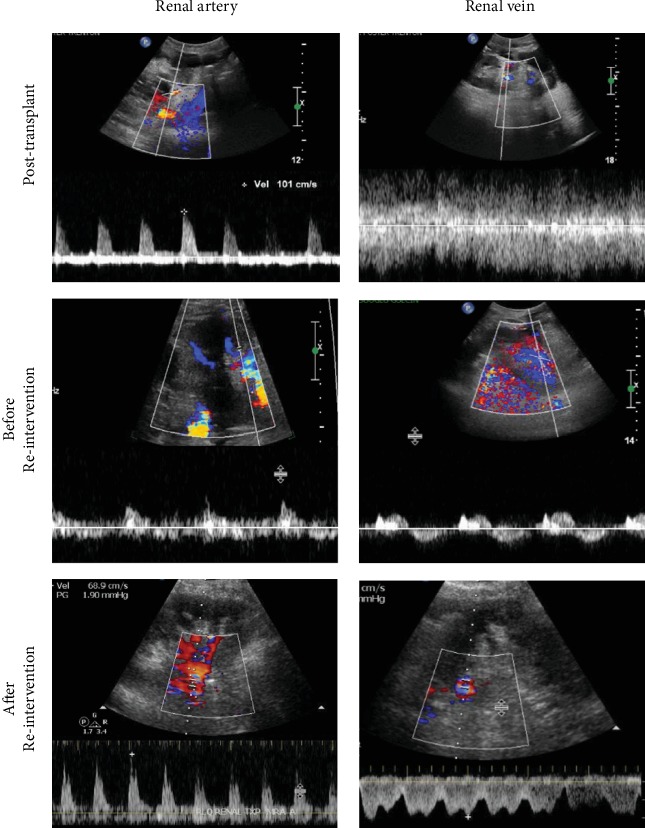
Ultrasound findings of the renal allograft vein and artery.
